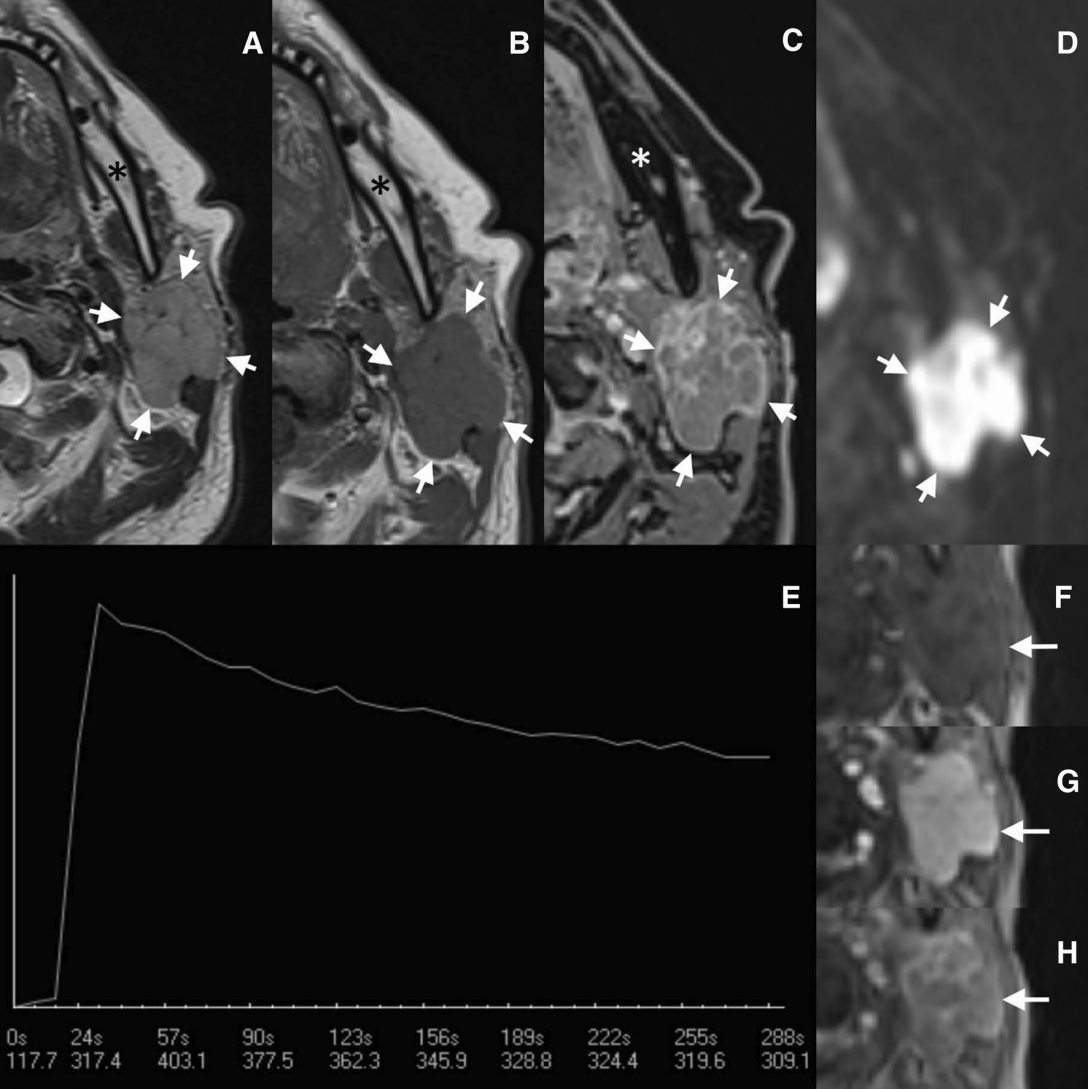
Magnetic resonance imaging in the axial plane of the left parotid region. In the turbo spin-echo sequence in T2-weighted image (a), the tumor (marked by the arrows) has intermediate signal intensity, observed in hypercellular lesions. In the turbo spin-echo sequence in T1-weighted image (b), the signal intensity is low. In the delayed contrast-enhanced gradient-echo T1-weighted image (c), the lesion shows heterogeneous and circumferential enhancement. In diffusion-weighted image (d) a marked diffusion restriction is observed (0.60–0.67 × 10^−3^ mm^2^/s), also typical for hypercellular lesions. The enhancement curve (e) shows the lesion’s signal intensity change after contrast agent administration on a timeline (expressed in seconds); early enhancement is visible with wash-out of the contrast agent (enhancement curve type B with wash-out ratio of 38%, typically seen in Warthin tumor). On pictures f to h, the subsequent phases of the dynamic examination are presented: pre-contrast image (f), early, strong, almost homogeneous enhancement of the lesion (g), and the last phase of the dynamic examination (h). Asterisk marks the mandible
